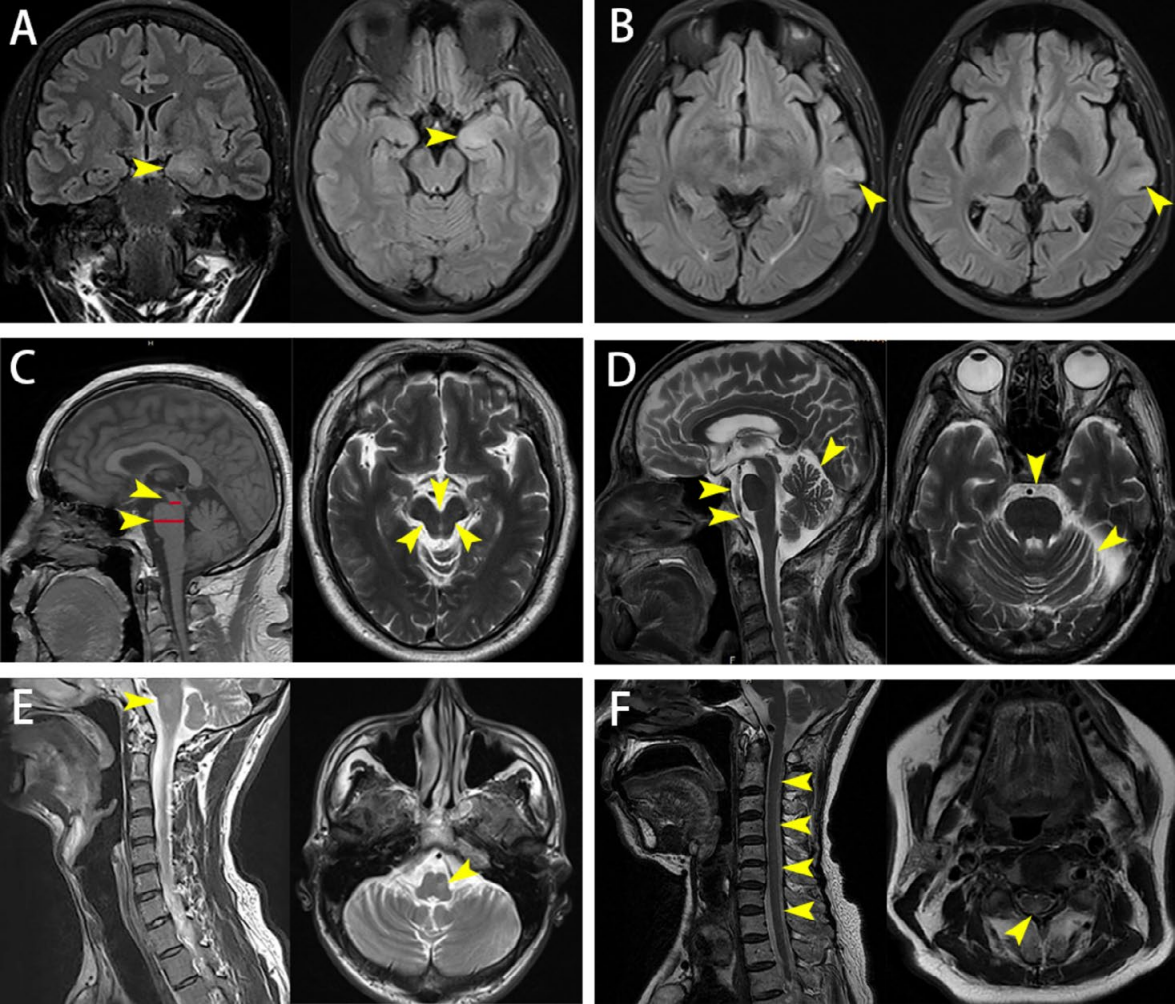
The distinctive MR imaging features of GAD65 neurological autoimmunity. (A) Fluid‐attenuated inversion recovery (FLAIR) imaging revealed swelling and hyperintensity in the left hippocampus and parahippocampal gyrus of a 33‐year‐old female patient with epilepsy. (B) Flair imaging demonstrated cortical thickening and hyperintensity in the left temporal lobe of a 24‐year‐old male patient with epilepsy. (C) T1‐weighted imaging (T1WI) and T2WI imaging revealed midbrain atrophy with the hummingbird sign in a 63‐year‐old male patient with a parkinsonian, showing the anteroposterior diameter ratio 0.41 of midbrain to pons. (D) T2WI imaging revealed widening of the pre‐pontine cistern and bilateral cerebellar subarachnoid spaces, along with mild atrophy of the left cerebellum, in a 77‐year‐old male patient with parkinsonian. (E) T2WI imaging revealed patchy abnormal signals in the left medulla of a 31‐year‐old male patient with overlap syndromes. (F) Cervical MRI showed dorsal strip‐like hyperintensity from C2 to T1 vertebral levels in a 69‐year‐old female with peripheral neuropathy.
